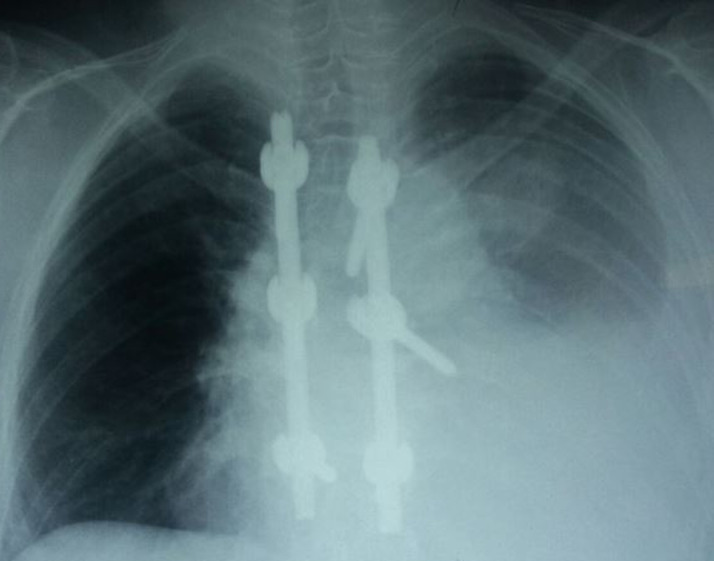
radiographie thoracique de face: épanchement pleural gauche, des images lacunaires au niveau de l’omoplate et les clavicules avec matériel d’ostéosynthèse au niveau du rachis dorsal
